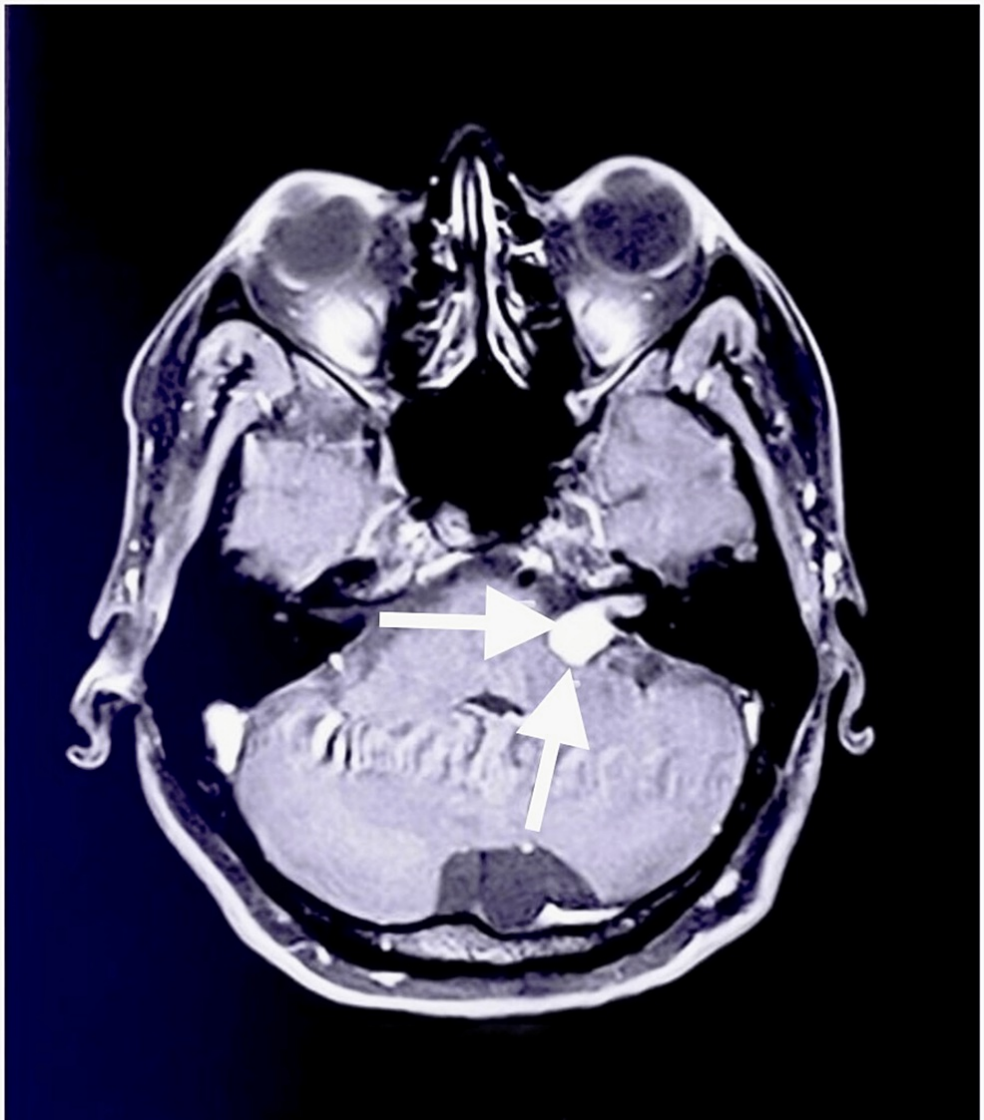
MRI of the brain showing the initial tumor (depicted by arrows). Intensely enhancing lesion in the left cerebellopontine angle with intracanalicular extension.
